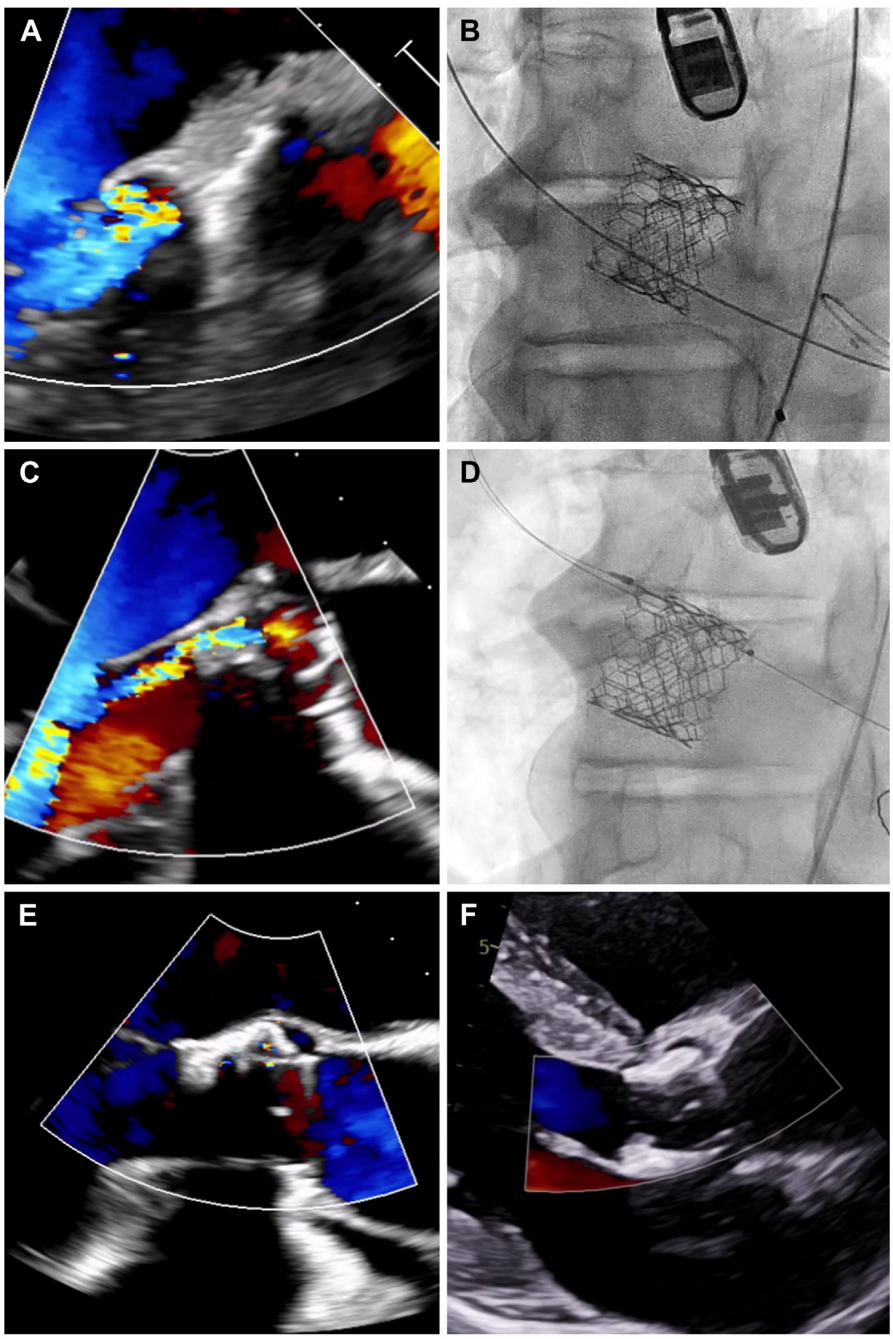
Case 3 Paravalvular Leak Evaluation and Final Result (A) Transesophageal echocardiographic evaluation of paravalvular leak after index transcatheter aortic valve replacement evidencing a mild paravalvular leak. (B) Final angiographic view after redo transcatheter aortic valve replacement in an attempt to resolve the paravalvular leak. (C) Transesophageal echocardiographic evaluation of a paravalvular leak after redo transcatheter aortic valve replacement evidencing a persistent significant paravalvular leak. (D) Angiographic result after percutaneous plugging. (E) Postprocedural transesophageal echocardiographic evaluation with only a trace paravalvular leak. (F) Follow-up transthoracic echocardiogram with no evidence of paravalvular leak.
